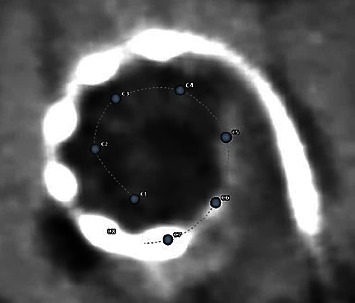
Implanted electrode array imaged via postoperative CT. The positions of the apical contacts are shown as numbers and dots after a 3D image reconstruction because not visible being on a different plane.
